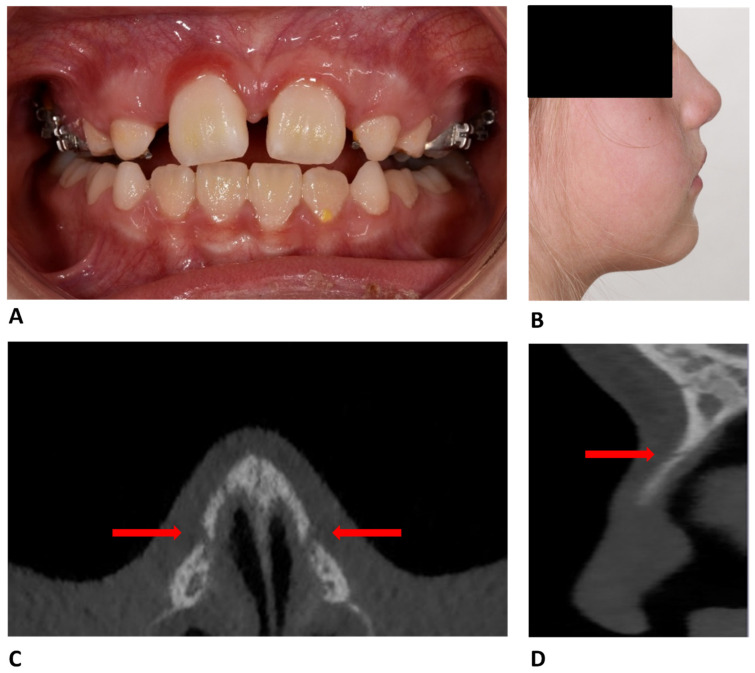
(A) Intraoral photograph at the initial visit. (B) Extraoral profile photograph. (C) CBCT axial slice showing bilateral discontinuity of the nasal bone. (D) CBCT sagittal slice showing discontinuity of the nasal bone.
